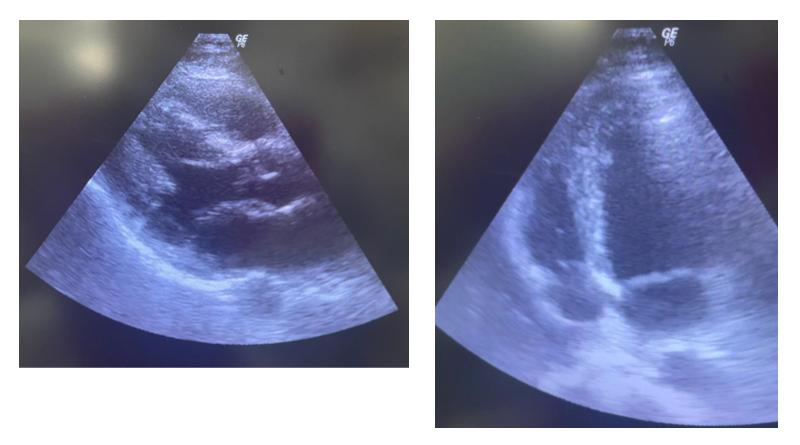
Transthoracic echocardiography with no direct signs of pulmonary arterial hypertension.
